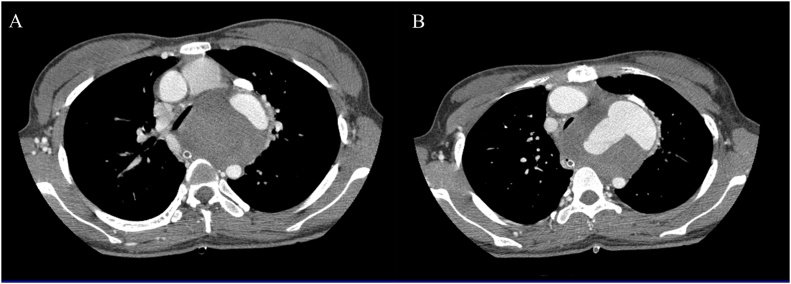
The image of aortic arch rupture by the chest computed tomography scanner. A, the size of aortic arch without hematoma was 2.1 × 4.18 cm, ascending aorta was 3.08 cm, descending aorta was 1.31 cm. B, the size of aortic arch without hematoma at rupture position was 4.68 × 6 cm, ascending aorta was 3.11 cm, descending aorta was 1.28 cm.
